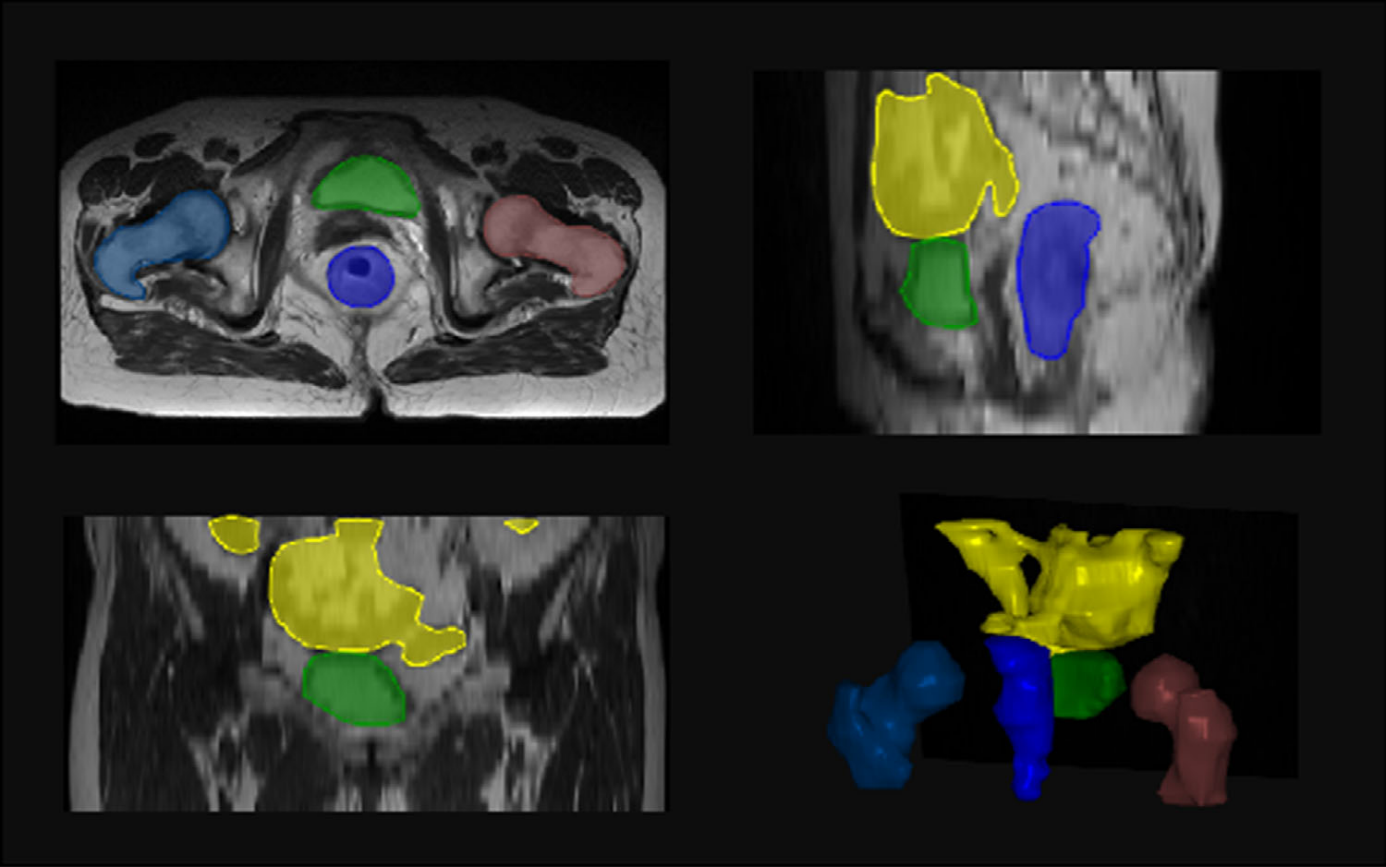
The OAR contours in MR image. Yellow: small intestine, green: bladder, blue: rectum, indigo: femoral head right and brown: femoral head left.
